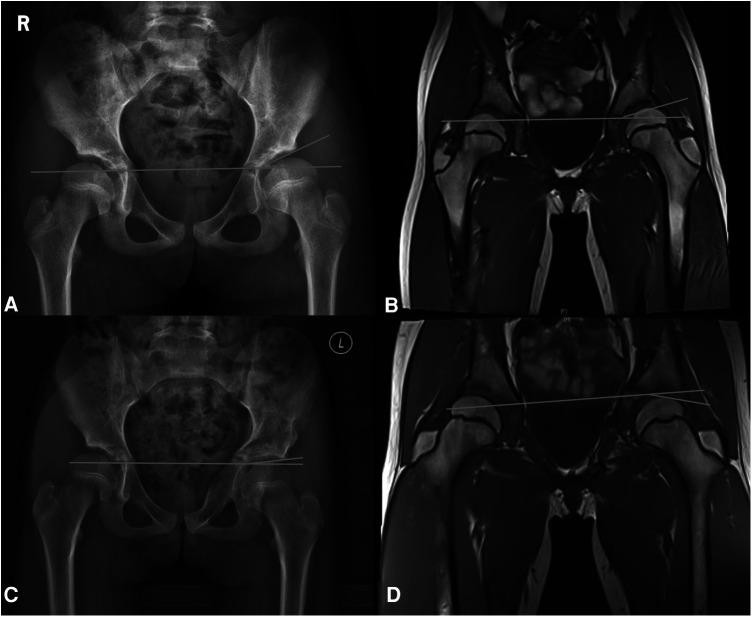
(A) A child 9 years old, previously treated conservatively with bilateral RHD. (B) An MRI of a 9-year-old child showing a left CAI of 16.1° and an AAR of 10.36. (C) An 11-year-old child, 2 years after the performance of Bernese triple pelvic osteotomy on the left side. (D) A postoperative MRI of a child with a left CAI of −14.3°.
